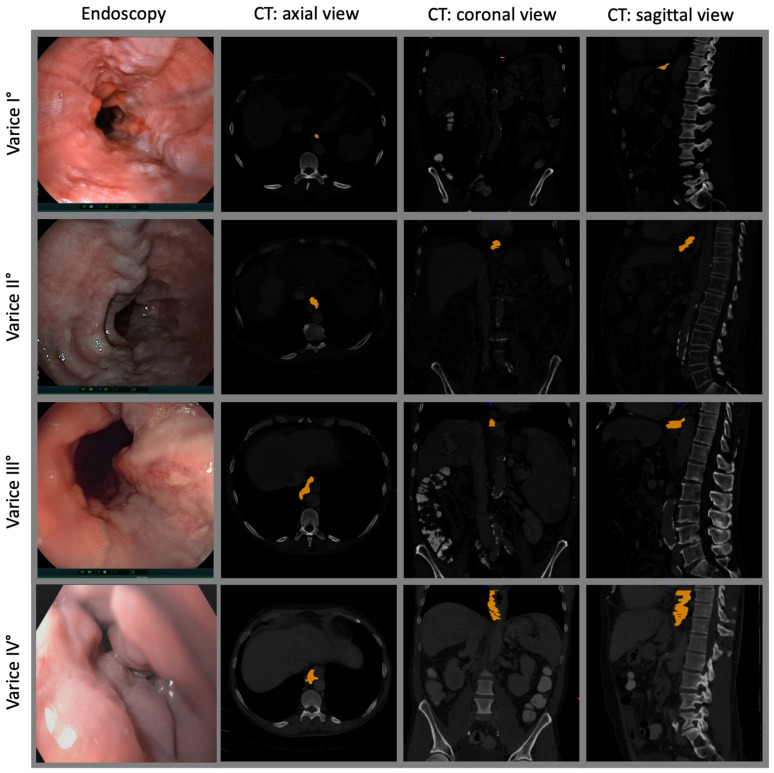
Examples of endoscopic and CT volumetry for variceal measurement.
